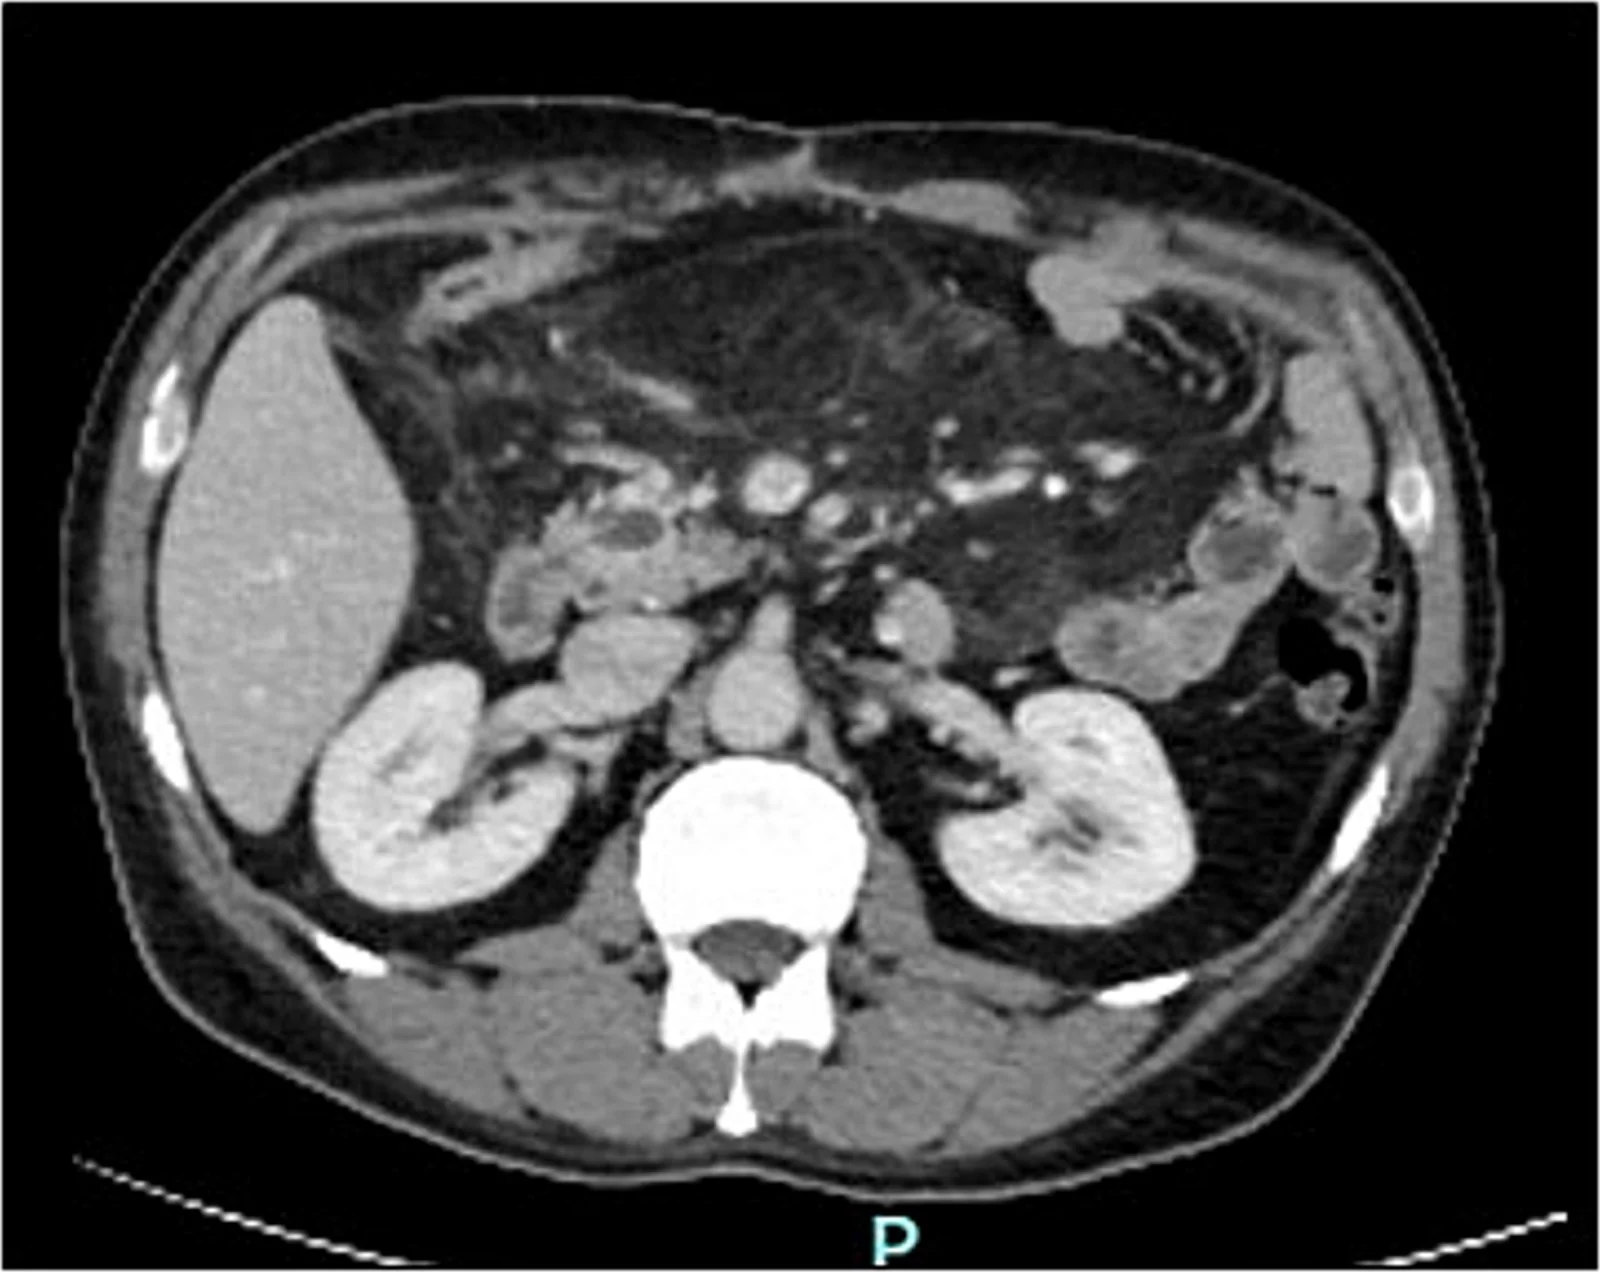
Computed tomography imaging of the patient in January 2020 demonstrating persistent mesenteric haziness compatible with ongoing sclerosing mesenteritis.
